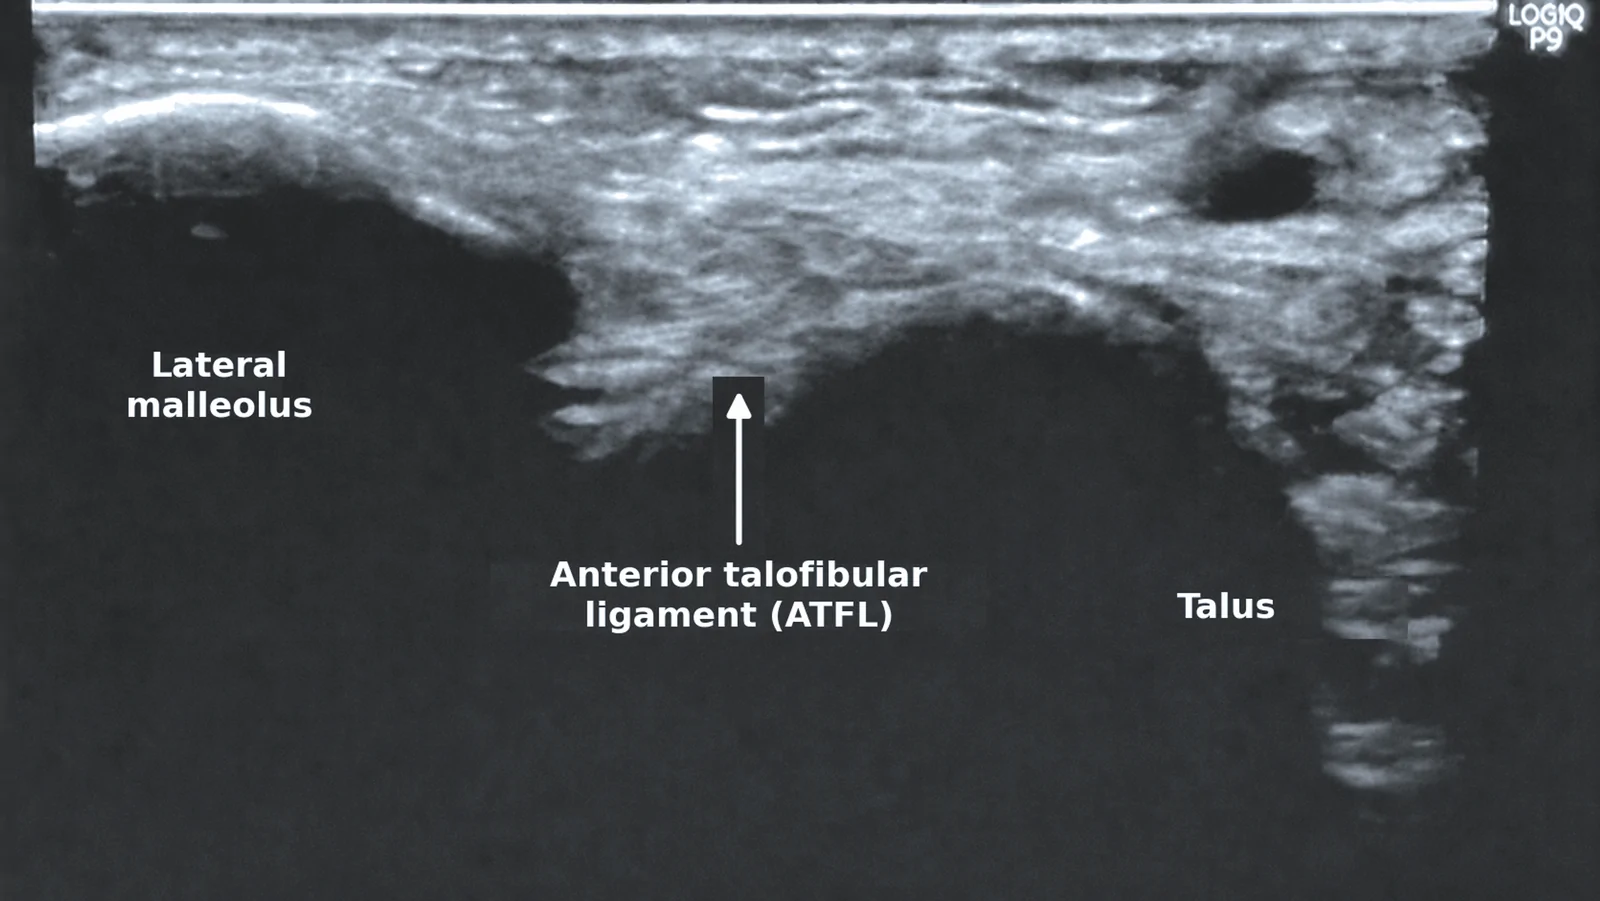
Ultrasonographic findings at initial assessment Static ultrasound image of the lateral ankle at initial assessment. The anterior talofibular ligament (ATFL) region is indicated by the arrow; its continuity was indistinct, a finding suggestive of lateral ligament injury. The lateral malleolus and talus are labeled for anatomical orientation. Patient identifiers were removed.
